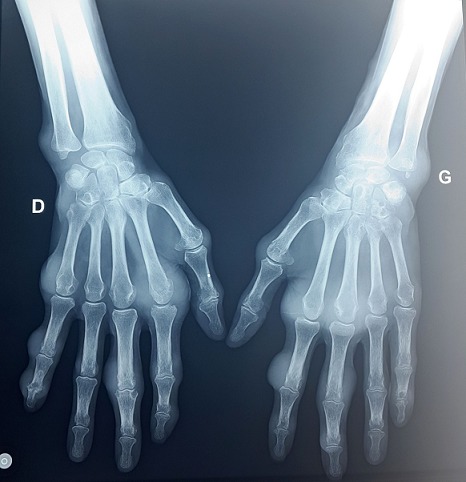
Radiography of both hands showing soft-tissue swelling and periarticular erosions in metacarpophalangeal and interphalangeal joints
